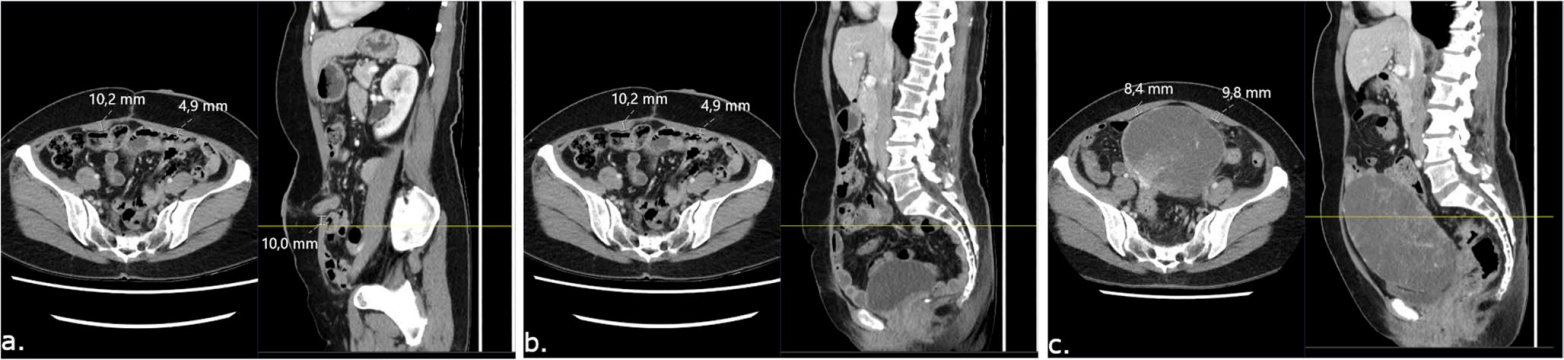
Abdominal rectus muscle (ARM) measurements on computerised tomography (CT) scans of rectal cancer patients subjected to tumour resection with permanent end colostomy. First, on postoperative CT (a.), the location of the stoma is identified, and ARM measurements are made on axial slices 1 cm caudal to the stoma aperture. On sagittal slices (b.) the craniocaudal level at which the measurements were made is then established in relation to fix structures such as the vertebral column. Then, on preoperative imaging (c.) the same craniocaudal level is identified and ARM measurements are made on axial slices the same way as in (a.)
